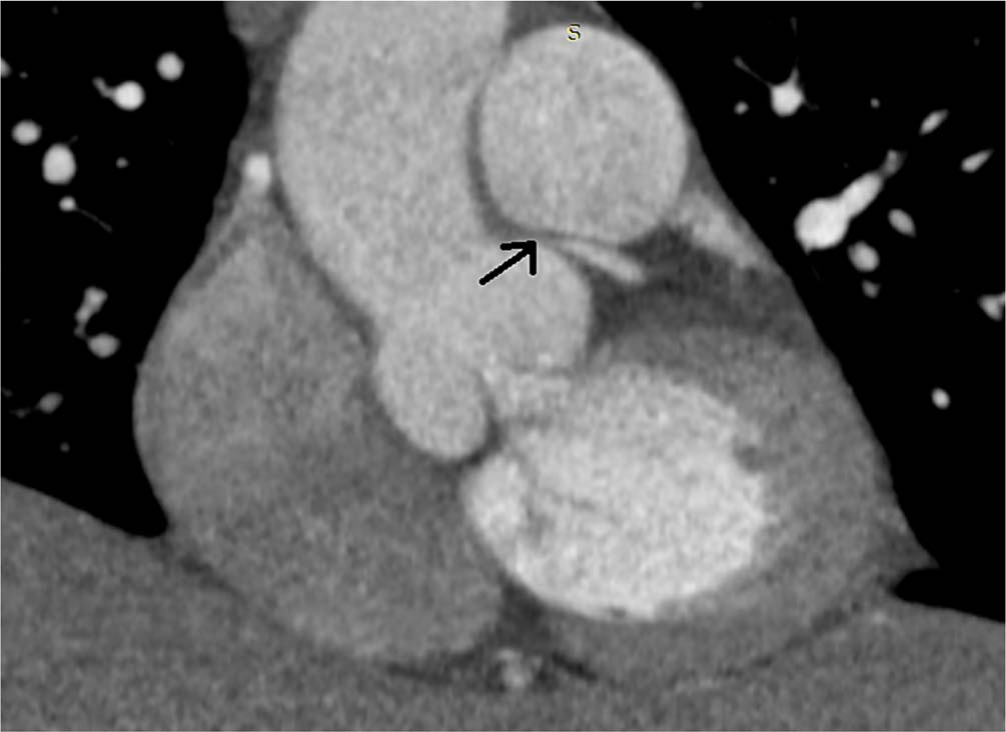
Preoperative multislice computed tomographic coronary angiography revealing sub-total occlusion of left main coronary artery, starting at the ostium and extending 5 mm distally (arrow)
